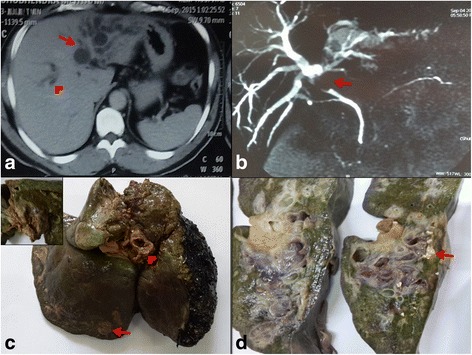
a CT scan showing dilatation of left intrahepatic bile duct (arrow), more than right (arrowhead); b MRCP showing filling defect (arrow) in common hepatic duct; c Left hepatectomy showing yellow-white nodules (arrow) with dilated, thickened hepatic ducts (arrowhead). Large thick walled bile duct showing necrotic material (Inset); d Multiple dilated, thick walled hepatic ducts, few studded with stones (arrow)
